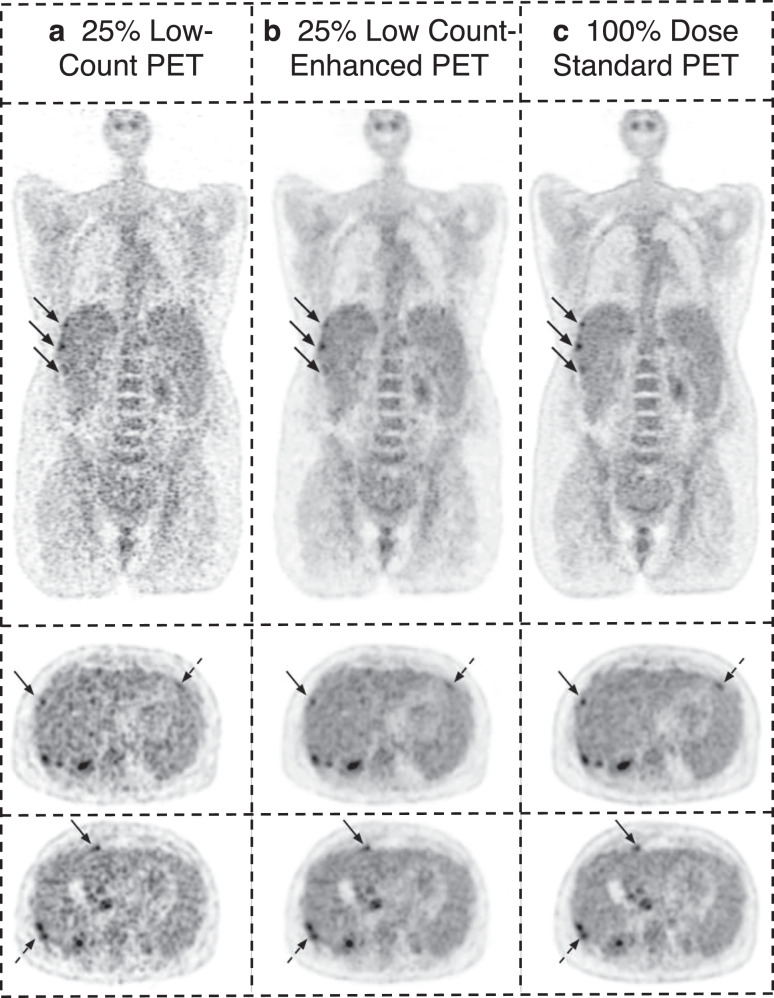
An example case showing the effectiveness of deep-learning enhancement of low-count PET in a 65-year-old female with BMI of 31 and an occult malignancy scanned on a GE Discovery MI scanner. A coronal slice and two axial slices through the liver are displayed for A 25% low-count, B 25% low-count-enhanced, and C standard PET images. The low-count PET images are noisy and lesions (arrows) are obscured by noise. The low-count-enhanced images are effectively denoised by the low-count-enhancement algorithm and the lesion conspicuity is similar to standard PET images. Note: SUV display scale is 0–7.
